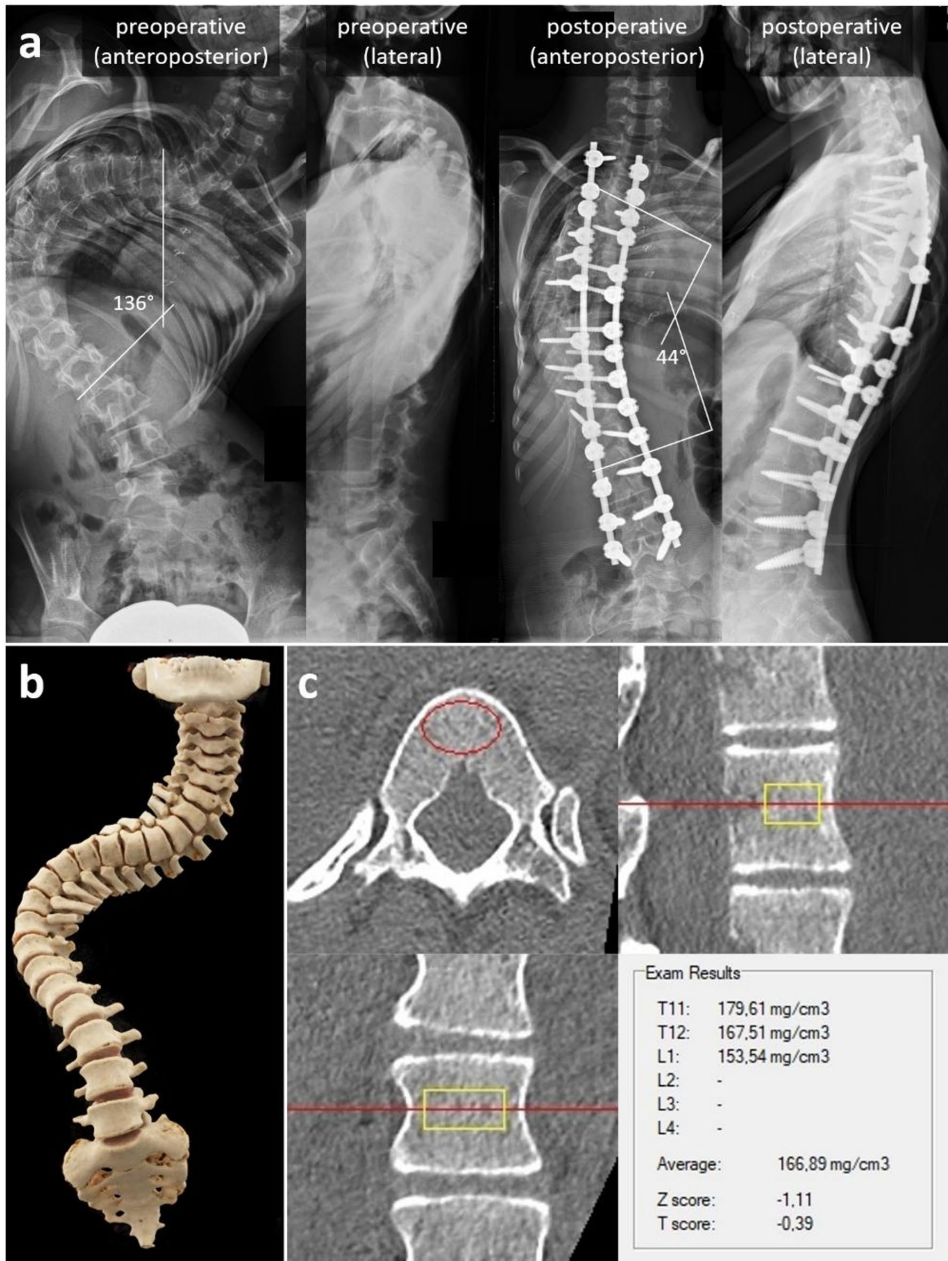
(a) Preoperative and postoperative anteroposterior and lateral sitting radiographs of a 13 years female patient with cerebral palsy, with correction of scoliosis from an angle of 136° to 44° after extensive spinal instrumentation and fusion from T (thoracic) 2 to L (lumbar) 5 vertebra, which equals to 68% scoliosis correction. (b) The spine model reconstructed from computed tomography data shows severity of spinal misalignment in all three dimensions. Therefore, in order to extract volumetric bone mineral density values (c), accurate alignment on all three anatomical planes is required for each vertebral body to be measured. Red line in sagittal and coronal plane represents the level of the cross-section seen in axial plane. The red circle in axial plane defines the circumference and yellow rectangles in sagittal and coronal planes define height, depth and width of the volume of the range of interest, in which bone mineral density is measured. This volume must be centered on the vertebral body and may only contain cancellous bone, leaving out neurovascular structures and cortical bone.
